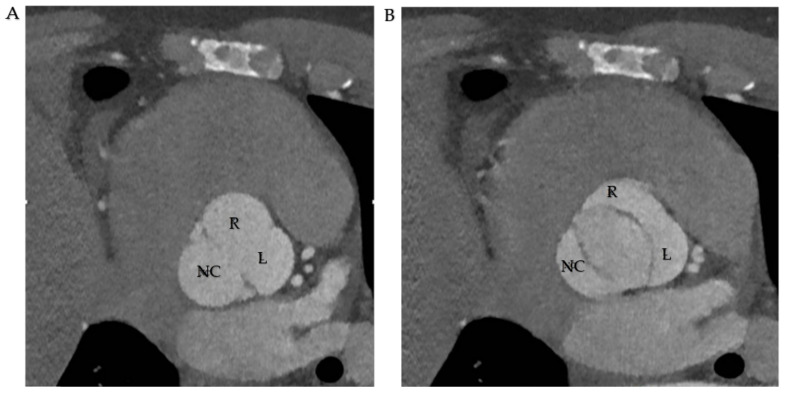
(A,B). CT scan in R-L cusp fusion. (A) Three sinuses are represented. (B). Opening mechanism in fusion pattern. Abbreviations; R, right coronary cusp; L, left coronary cusp; NC, non-coronary cusp.
